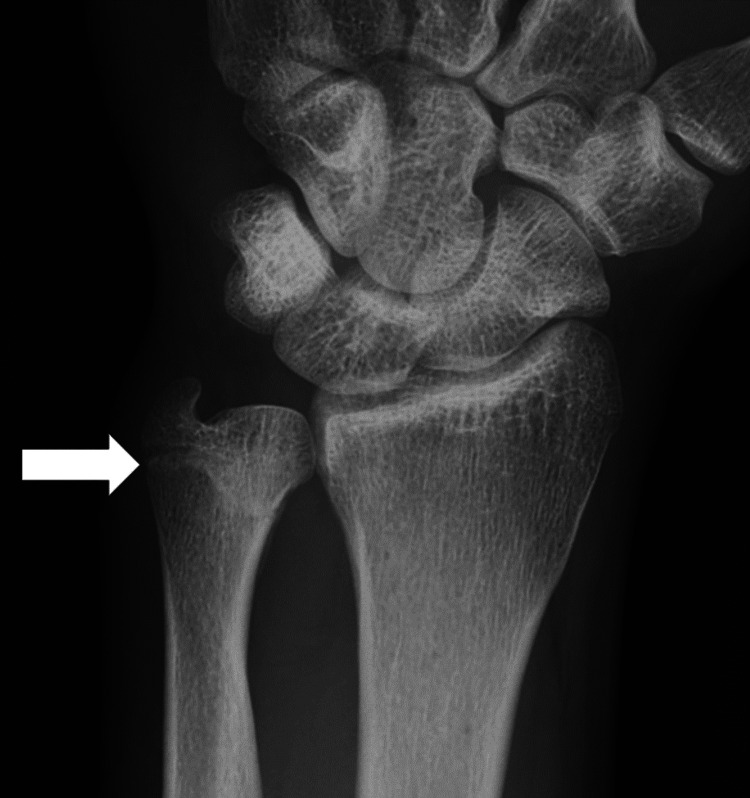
X-ray shows a fracture at the distal end of the ulna.
